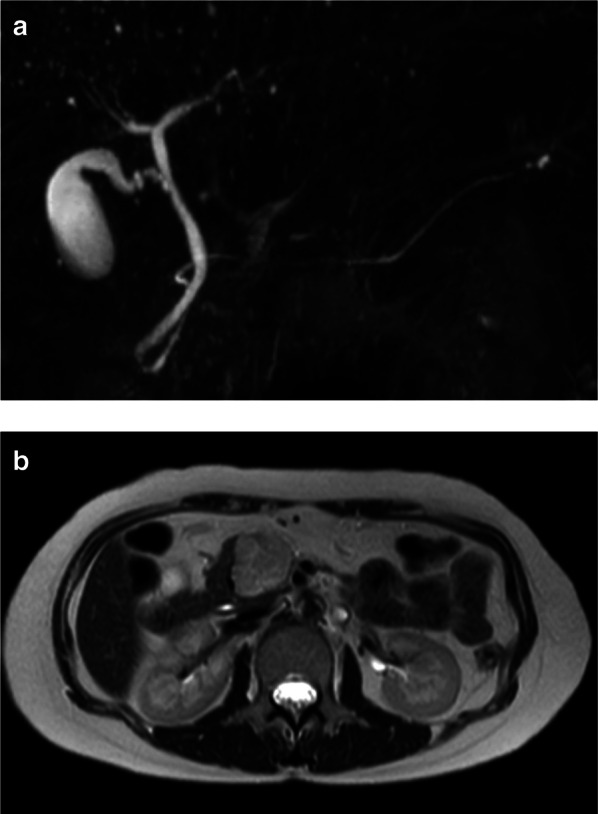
MR images. a Magnetic resonance cholangiopancreatography (MRCP) demonstrating an intact main pancreatic duct. b T2-weighted MR highlighting the tumor as a high signal area
